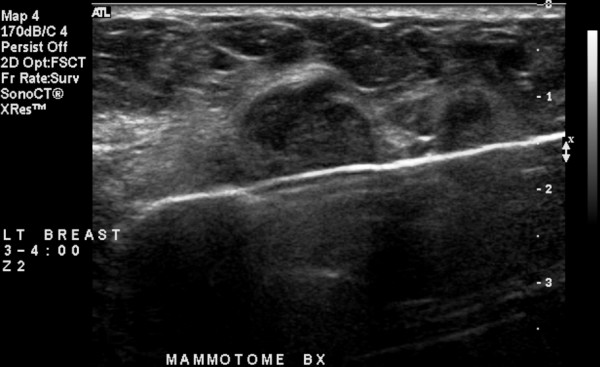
Ultrasound image in longitudinal axis showing the 8-gauge vacuum-assisted device positioned just beneath the ultrasound lesion once the cutter blade has been fully activated across the aperture of the tissue collection chamber.
